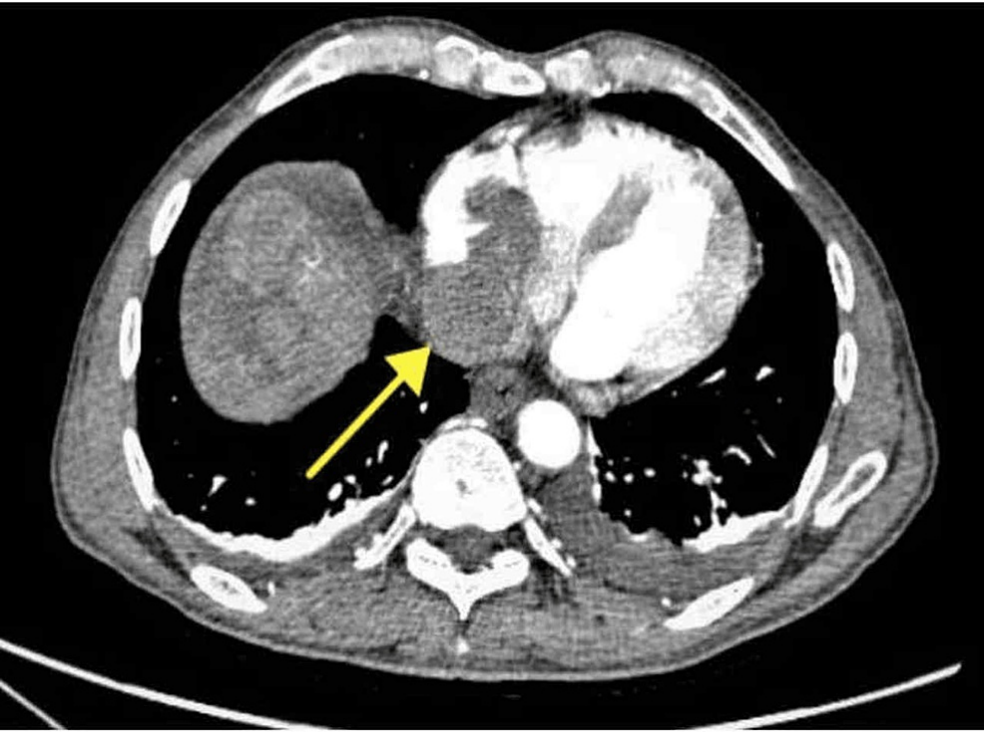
Dynamic hepatic CT scan axial section in arterial phase: included section of the chest at the level of the heart reveals a well-defined hypodense filling defect extending from the IVC in the right atrium (arrow) CT: computed tomography, IVC: inferior vena cava
